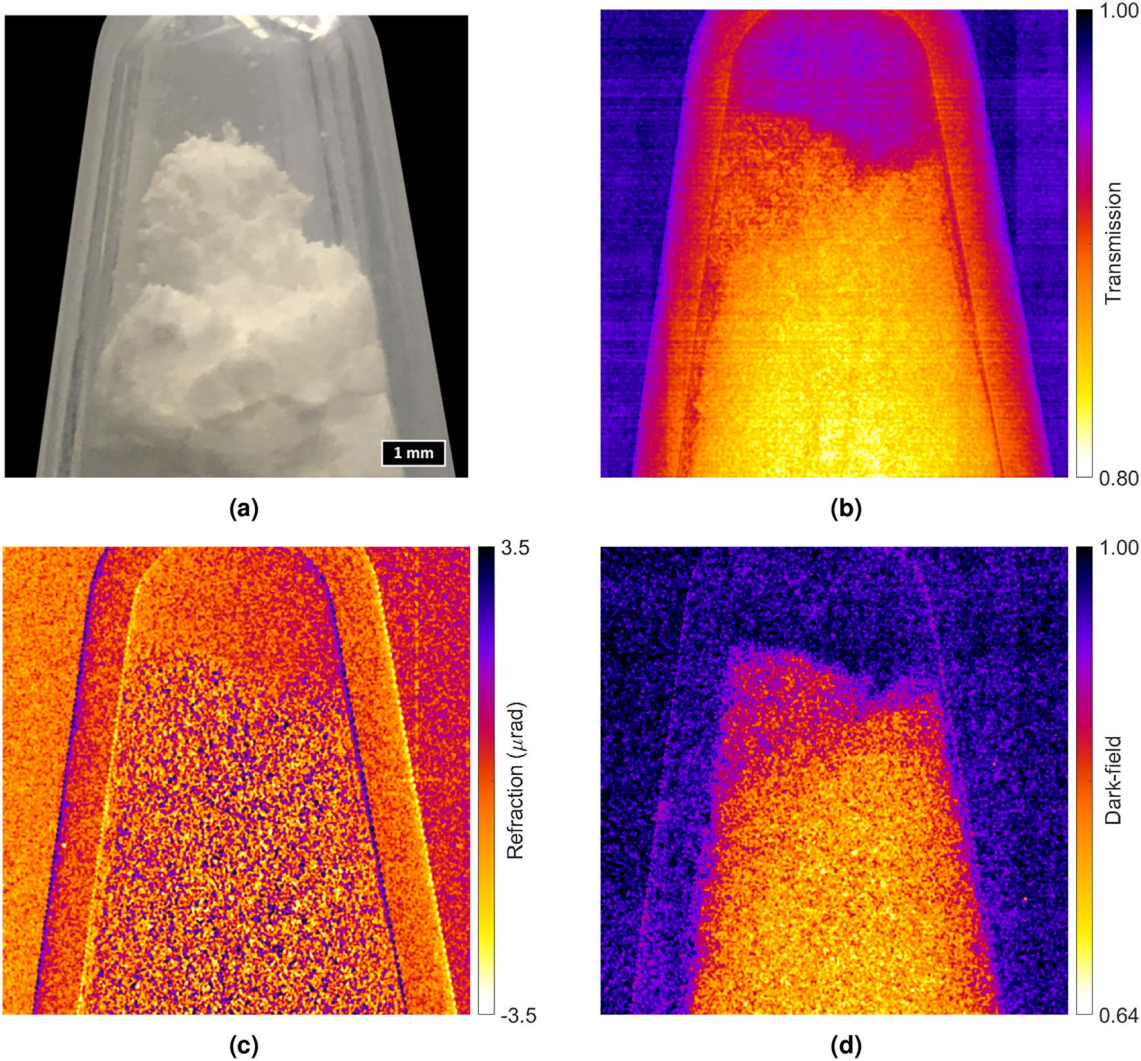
(a) A centrifuge tube filled with sugar powder as the sample of interest. (b) Transmission, (c) refraction, and (d) dark-field images retrieved from a single-exposure X-ray phase-contrast image taken at 60 $$\text{kV}_p$$ of the sample—images are represented based on a false color tone for better representation. As evident in the three contrast images, the grating artifacts do not show up in the final processed images.
